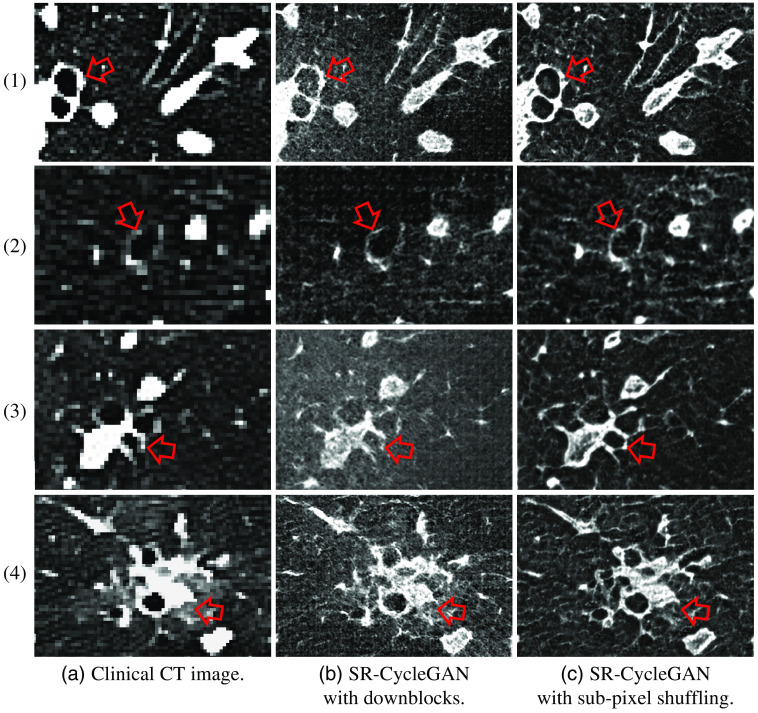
Comparison of SR-CycleGAN before/after removing downblocks and adding sub-pixel shuffling layers. Rows (1), (2), and (3) are CT images of the bronchus and blood vessel region. Row (4) has CT images of the tumor and bronchus region. Column (a) are clinical CT images. Column (b) and (c) are results of “SR-CycleGAN with downblocks” and “SR-CycleGAN with sub-pixel shuffling” respectively. After removing downblocks and adding sub-pixel shuffling layers, SR-CycleGAN performed better qualitatively. As indicated by the red arrows, results of SR-CycleGAN with downblocks (SR-CycleGAN with downblocks and before adding sub-pixel shuffling layers) have many artifacts, and the edges of the bronchus and blood vessels look discontinuous. On the other hand, these defects do not appear in the results of SR-CycleGAN.
